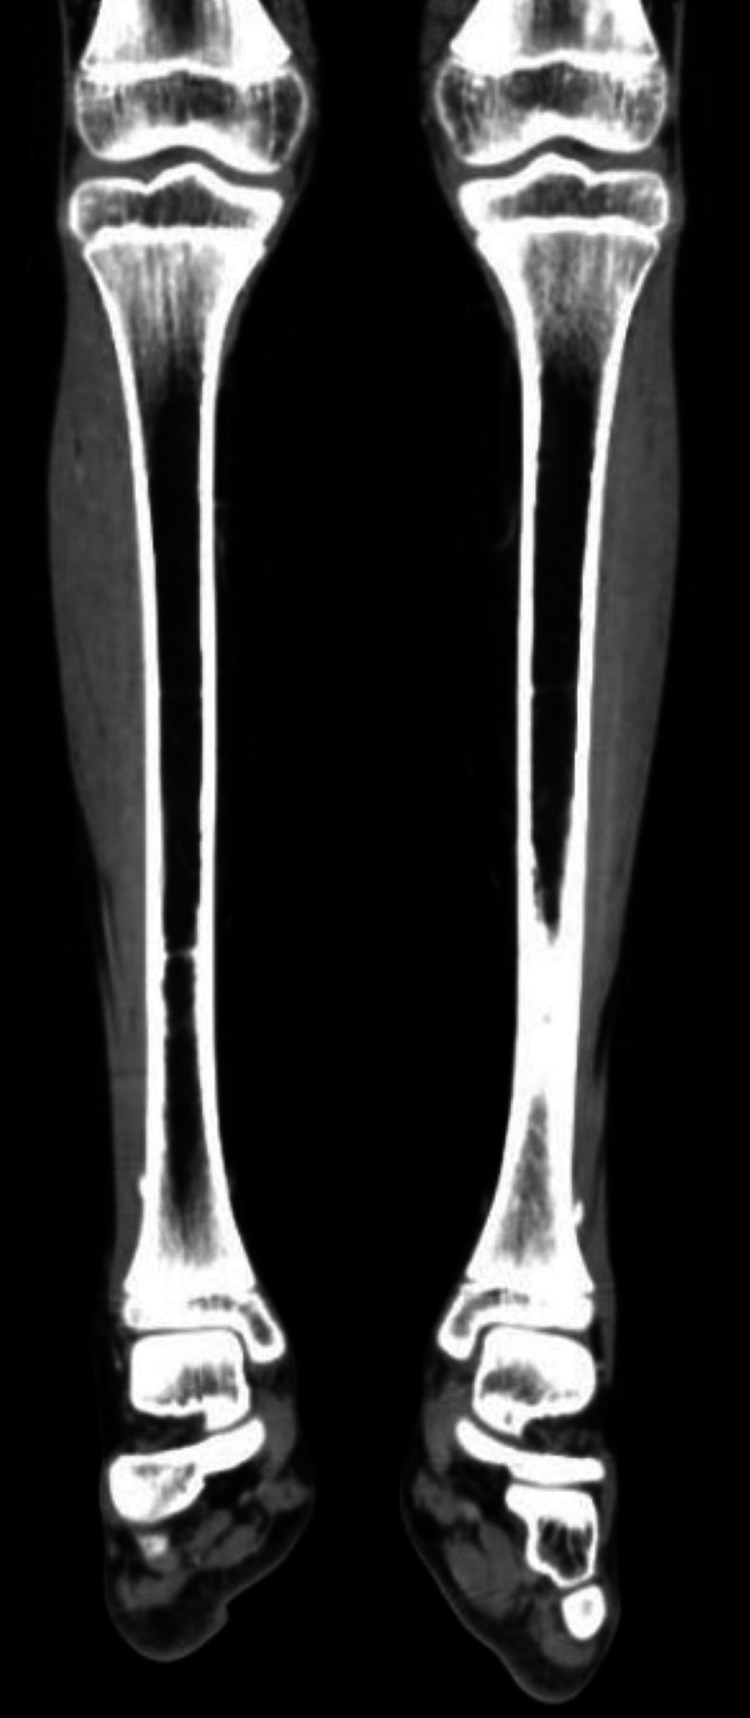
CT lower limb prior to fibula flap harvest, showing normal subtalar joint angulation.
